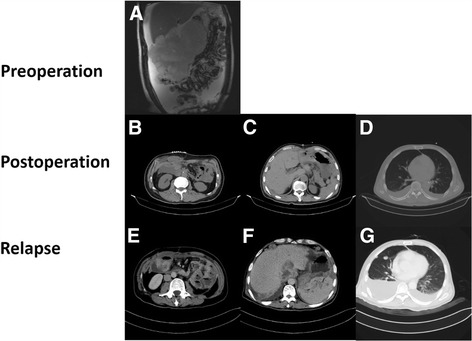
a. Preoperative abdominal MRI demonstrated a solid mass with an irregular lobulated configuration located in the right upper quadrant with a diameter of 20 cm (*blue arrow*). c, e, g. Patient was suffering from rapid recurrence with an extensive metastatic lesion in the abdominal cavity. b, d, f. The tumor was radically resected and the corresponding CT radiograph showed no lesions
